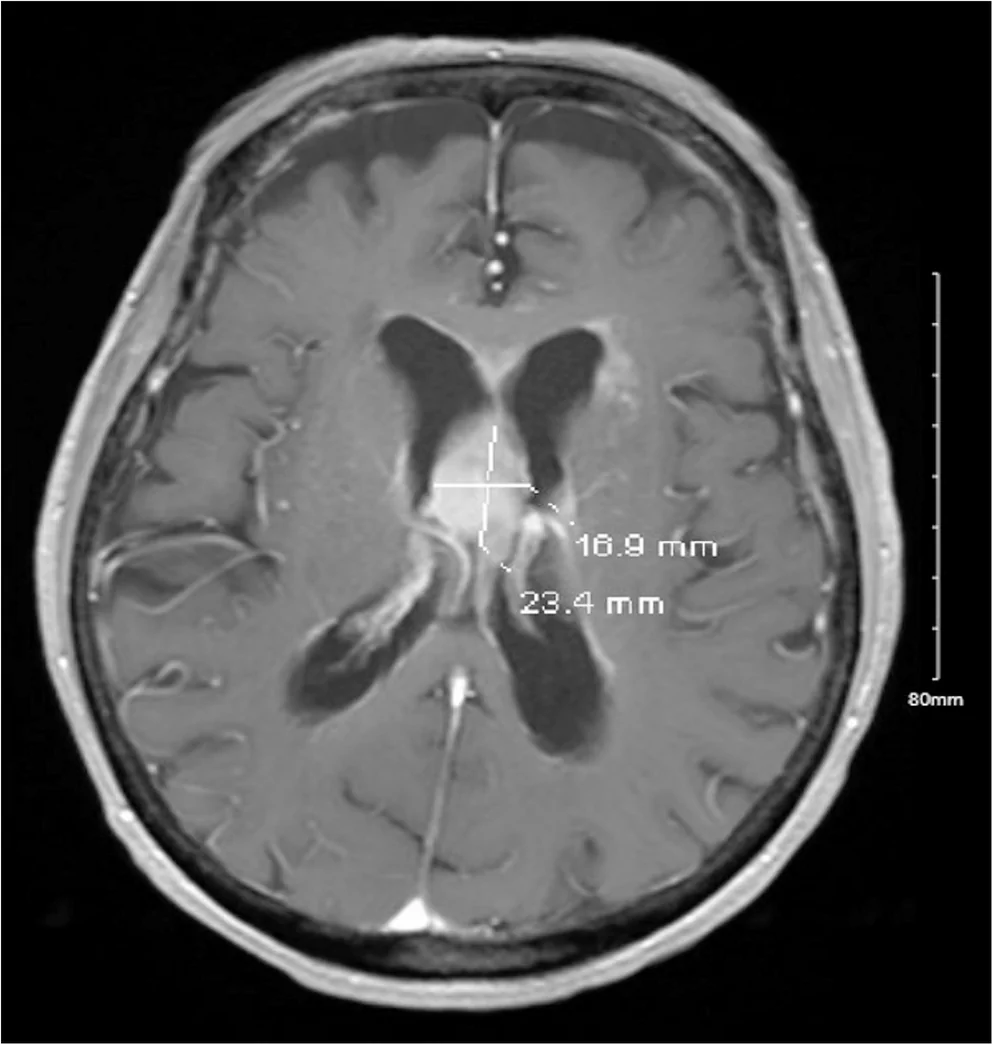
T1-weighted MRI showing a 2.3 × 1.7 × 2.4 cm enhancing tumor along the septum pellucidum extending between the frontal horns of the lateral ventricles with abnormal interventricular enhancement
